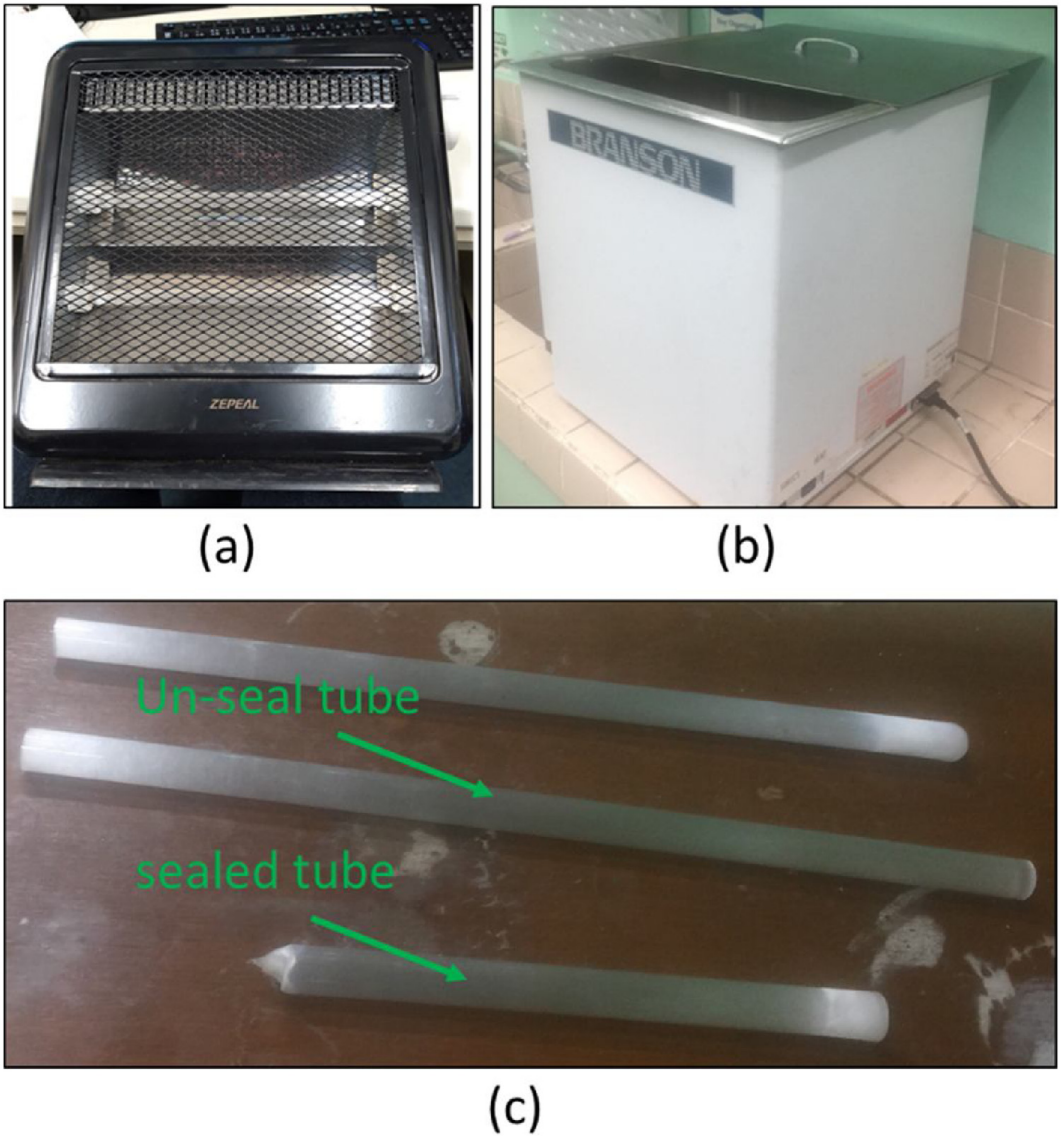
(a) Heating unit with silica tube inside, (b) ultrasonic cleaner, and (c) un-seal tube and sealed tubes.
